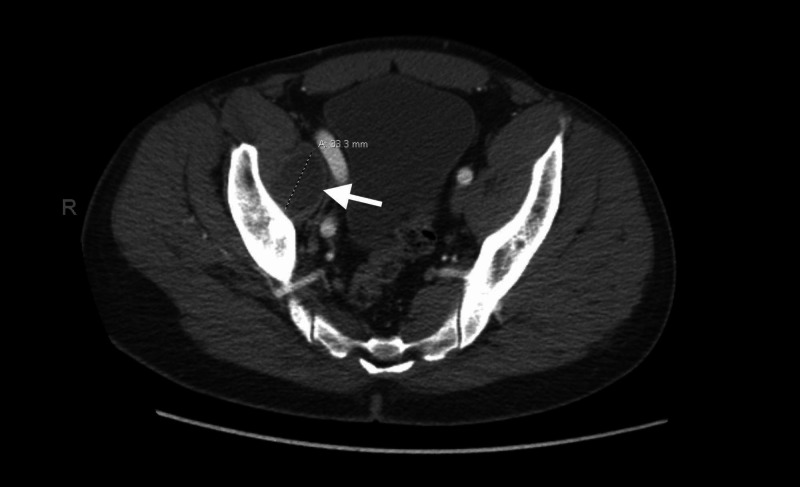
CT scan of the abdomen and pelvis. A well-circumscribed low-density collection is noted in the right psoas muscle, which measures 3.5 x 3.2 cm.
